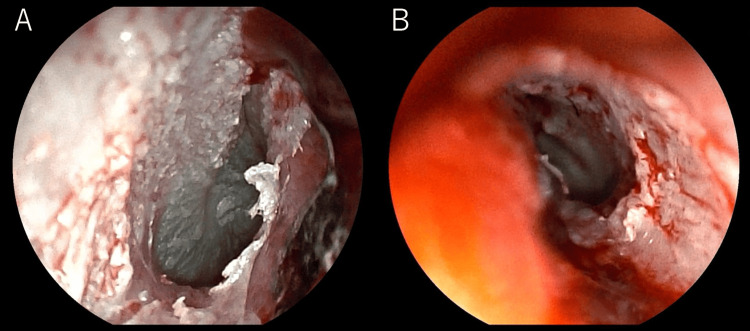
Otoscopic images of both ears after hematoma removal (A) Right external auditory canal. (B) Left external auditory canal. Both sides show edematous canal walls and mucosal injury with exposed tympanic membranes. These findings correlate with CT-demonstrated anterior external auditory canal wall fractures and otorrhagia.
